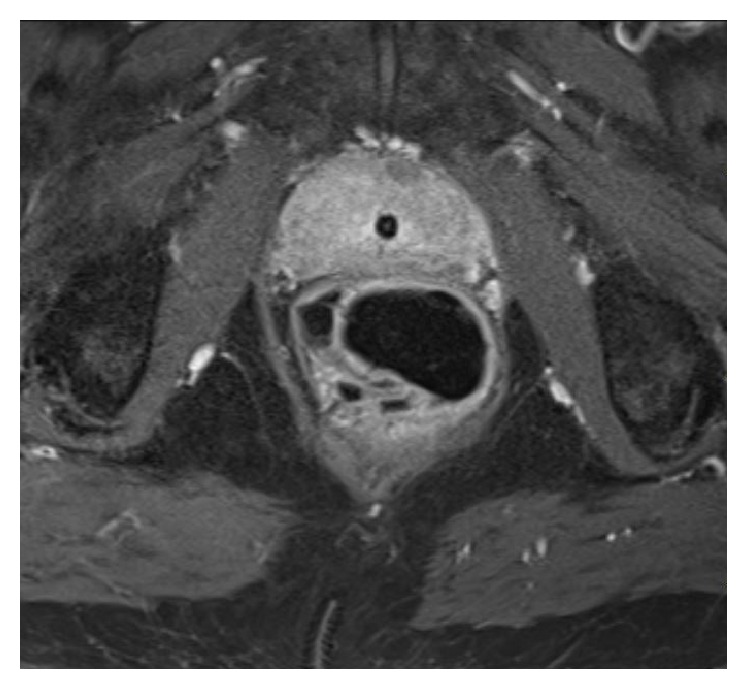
MRI showing anatomical changes of the rectum.
